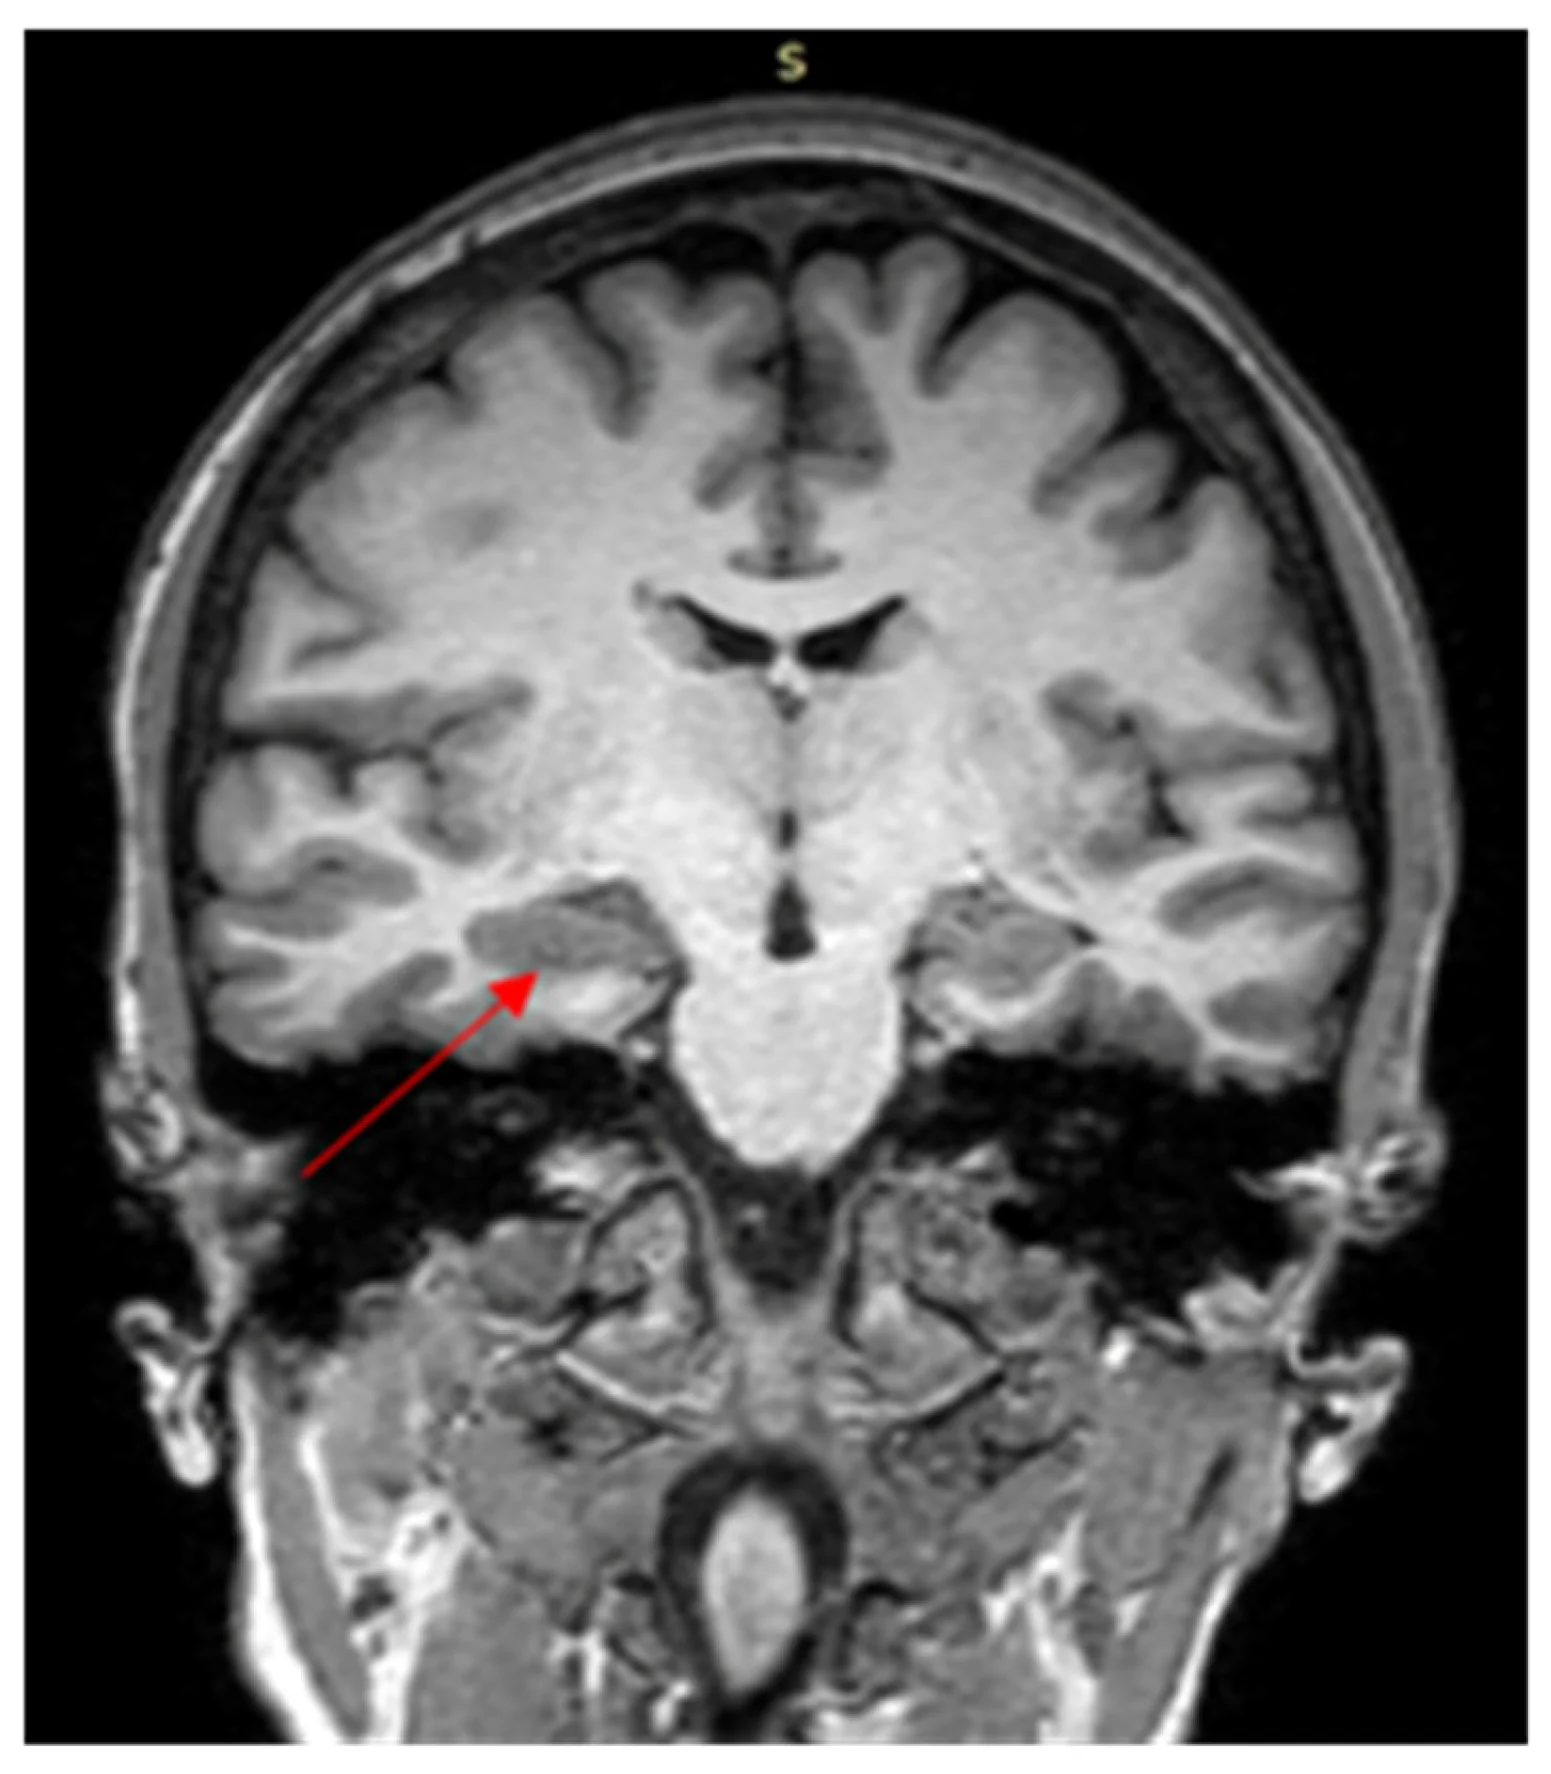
Whole-brain coronal T2-FLAIR MRI demonstrating the right hippocampal lesion (arrow).
